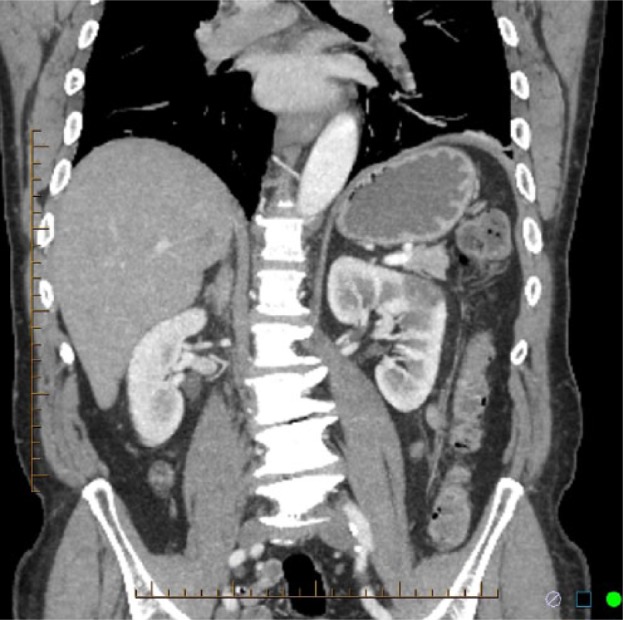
CT Scan of abdomen and pelvis showing wedge shaped non enhancing defect involving left inter polar region suggesting renal infarction.
